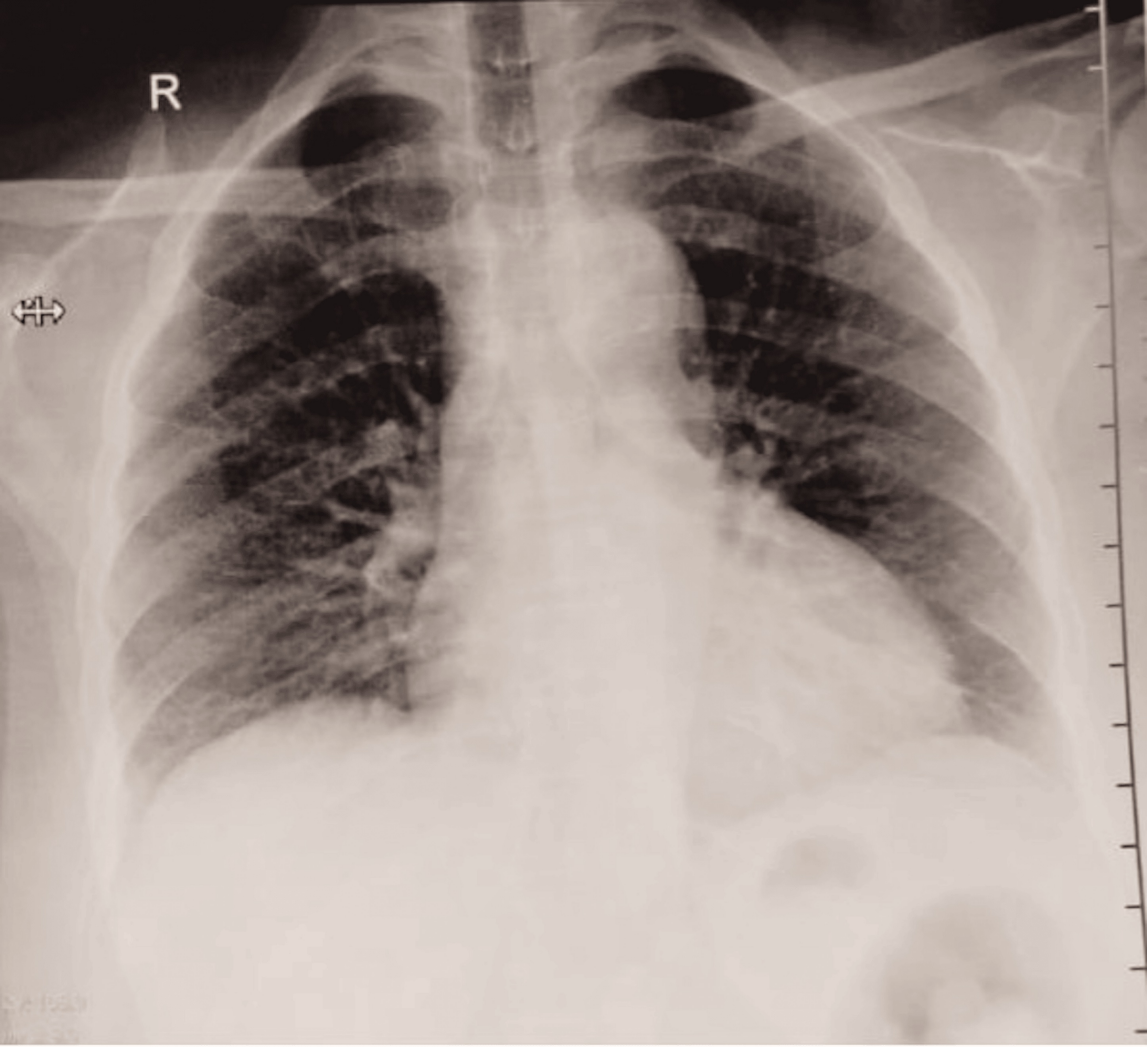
Chest X-ray posteroanterior view, showing no obvious abnormality
